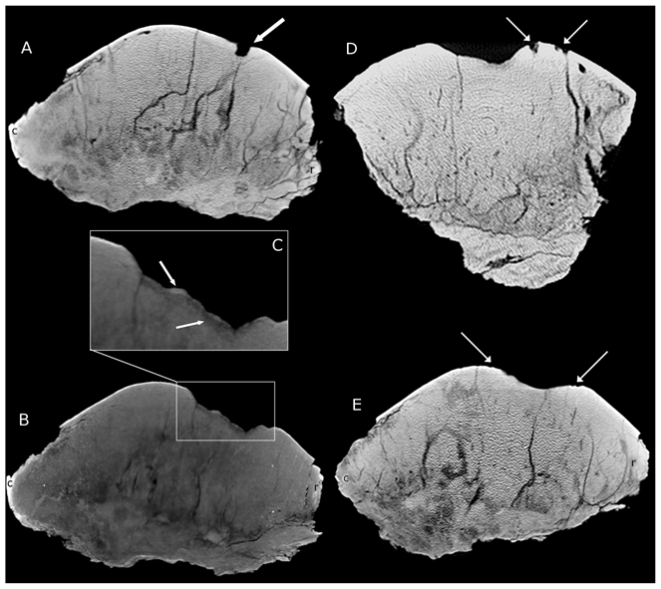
CT images of BMR P2001.4.5. A. Sagittal image showing a focal, deep penetrating lesion in the dorsal surface. B. Sagittal MIP (maximum intensity projection) reformatted image of the irregular surface of the large depressions. C, The surface of the depression possesses a high-density surface above a lower density band. D, Coronal, and E, sagittal images of the peripheral lesions at the margin of the larger central pathologies on the dorsal surface. r = rostral, c = caudal.
